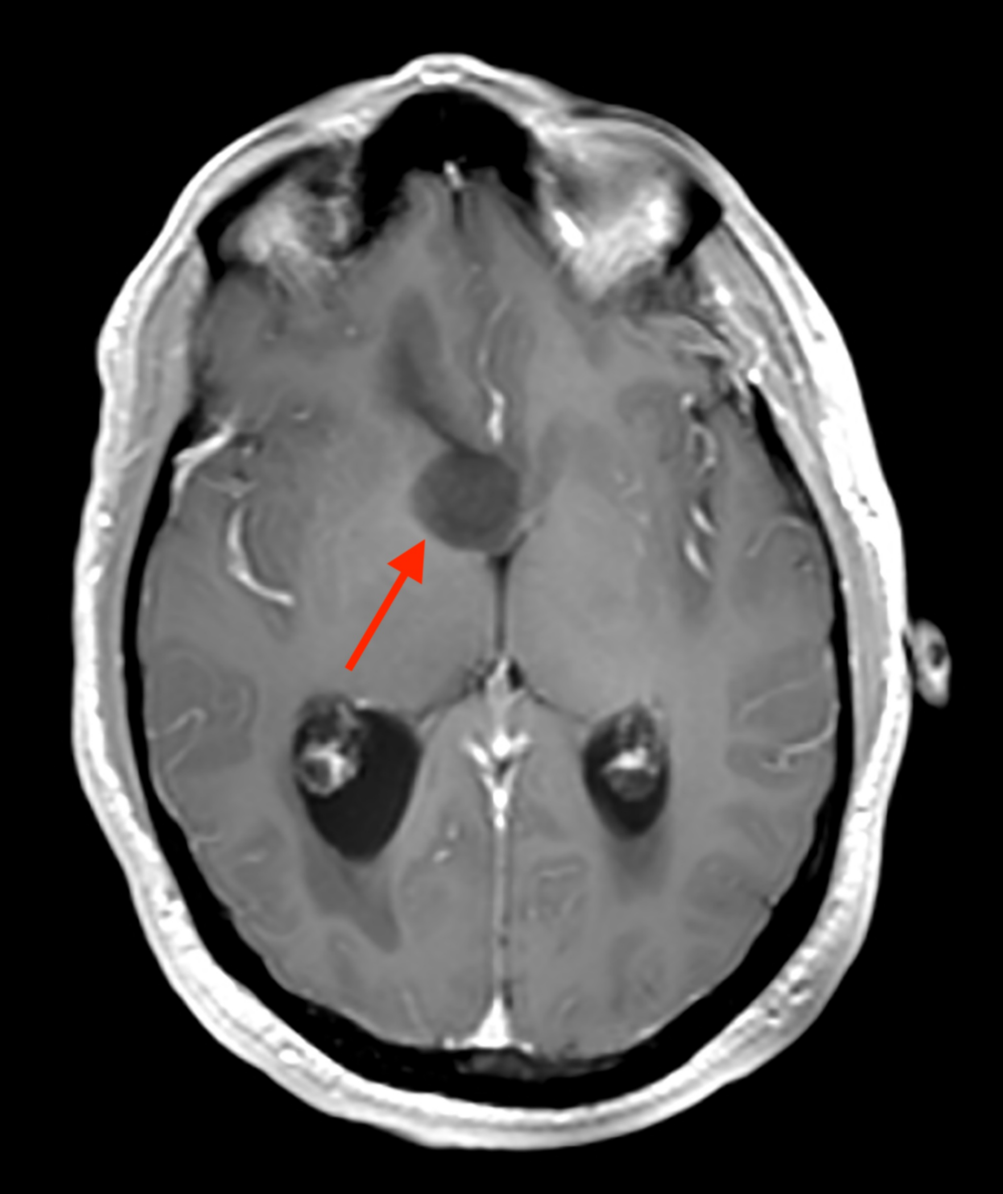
Post-contrast axial T1 weighted MRI with the arrow indicating foramen of Monro. The lesion is non-contrast enhancing, a feature not typically seen in this neoplasm.
